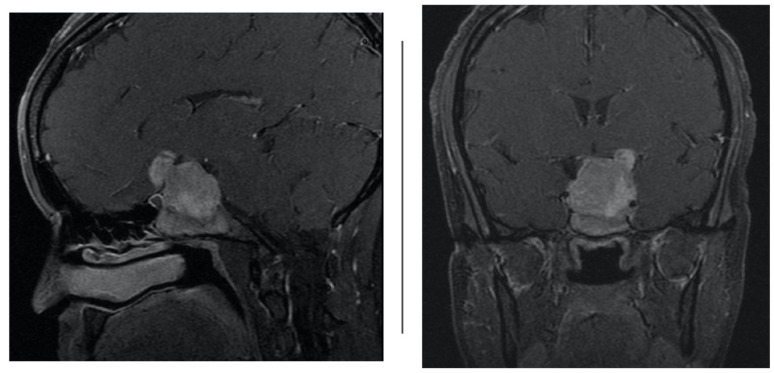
Sagittal and coronal T1 contrast-enhanced weighted magnetic resonance imaging (MRI) demonstrating large sellar and suprasellar pituitary macroadenoma extending into the cavernous sinus.
